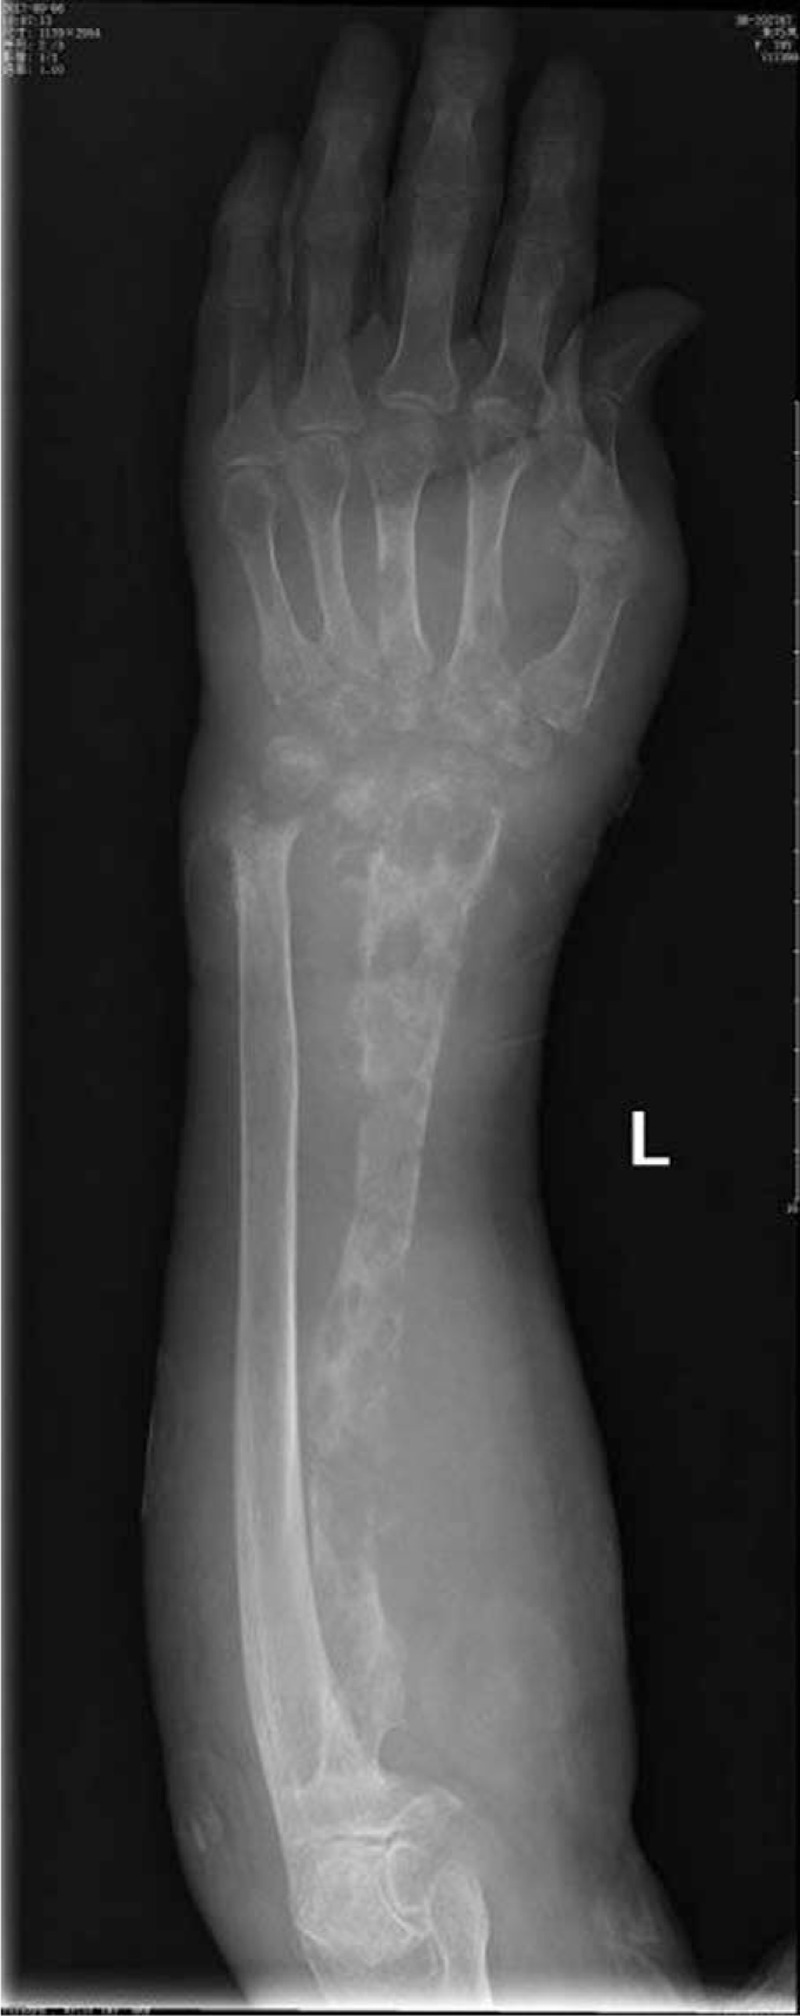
We could see radius, ulna, and carpal were eroded and disappeared in x-ray.
